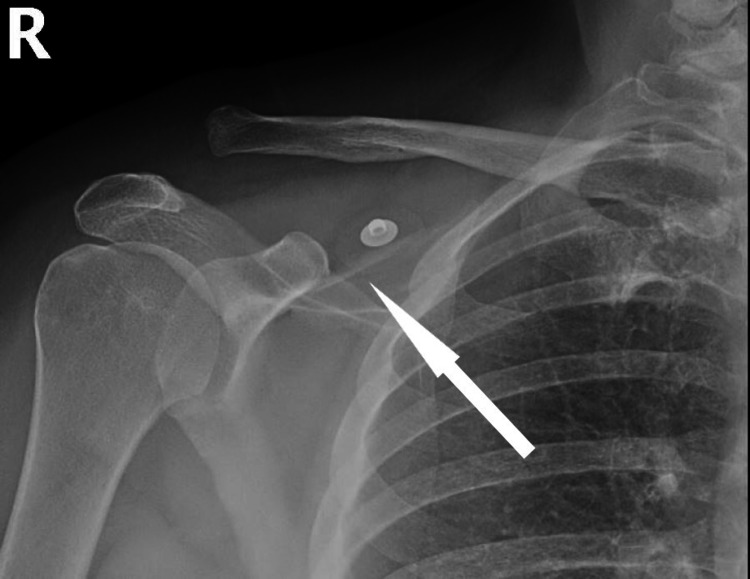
Injury anterior-posterior (AP) X-rays of the patient’s right shoulder at presentation
